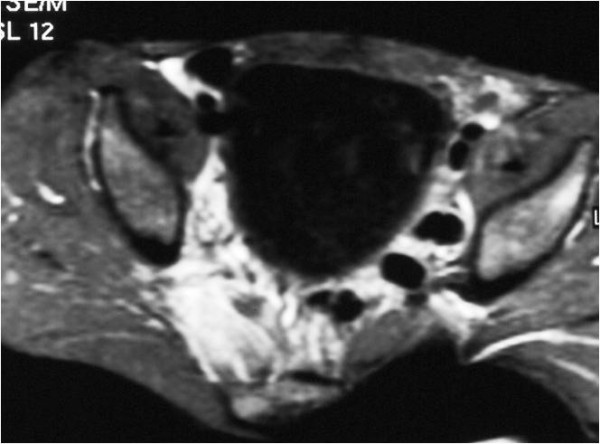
Axial spin-echo T1-weighted image with fat suppression with intravenous gadolinium contrast injection; MRI scan taken at the time of antibiotic treatment initiation. The right piriformis muscle enhances avidly after intravenous gadolinium, due to inflammation. Inflammatory changes are also depicted in the adjacent soft tissues of the pelvis.
